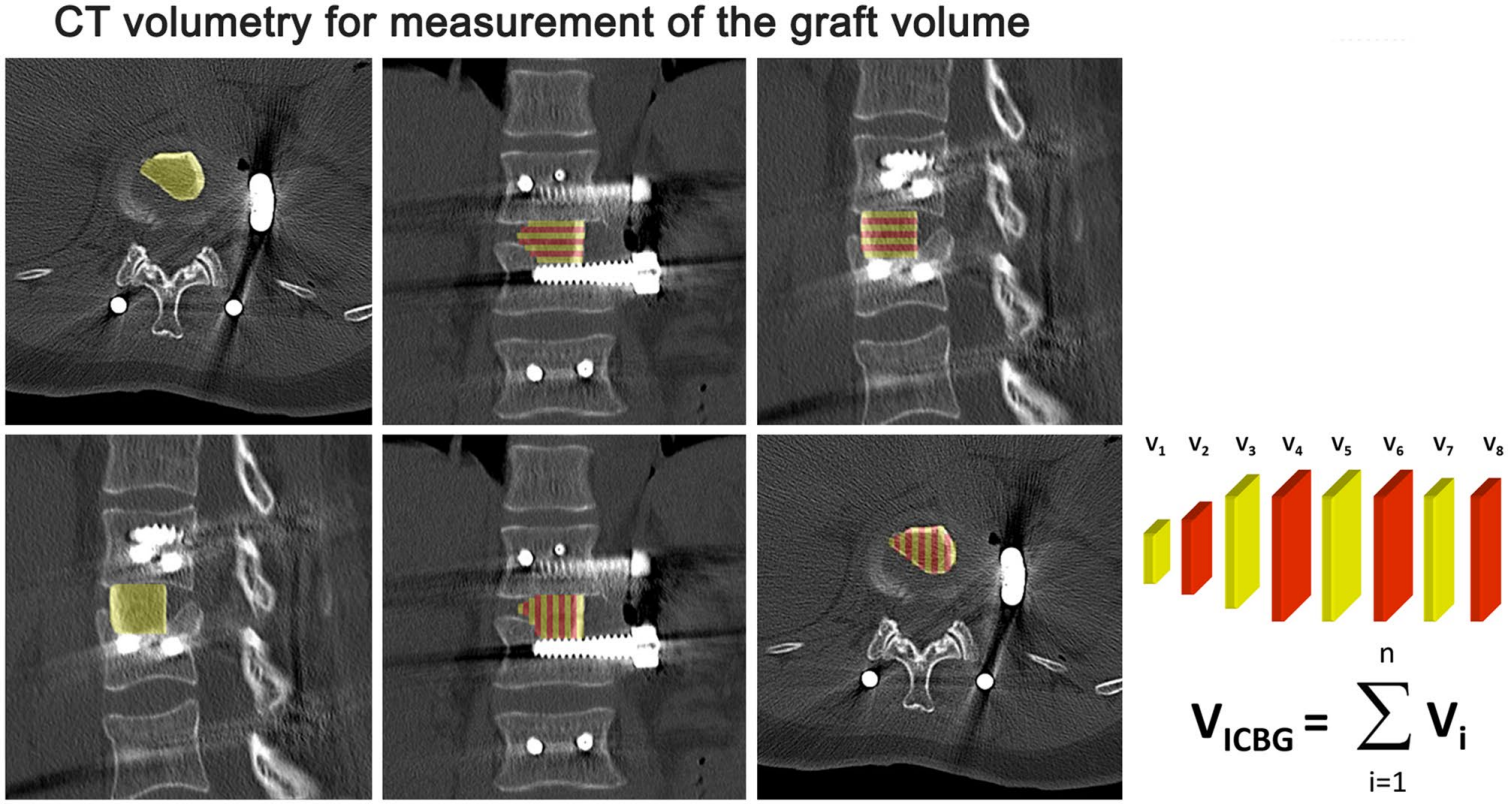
CT volumetry for estimation of the graft volume (ICBG). The CT scan demonstrates how the volume was measured in all three plains.
